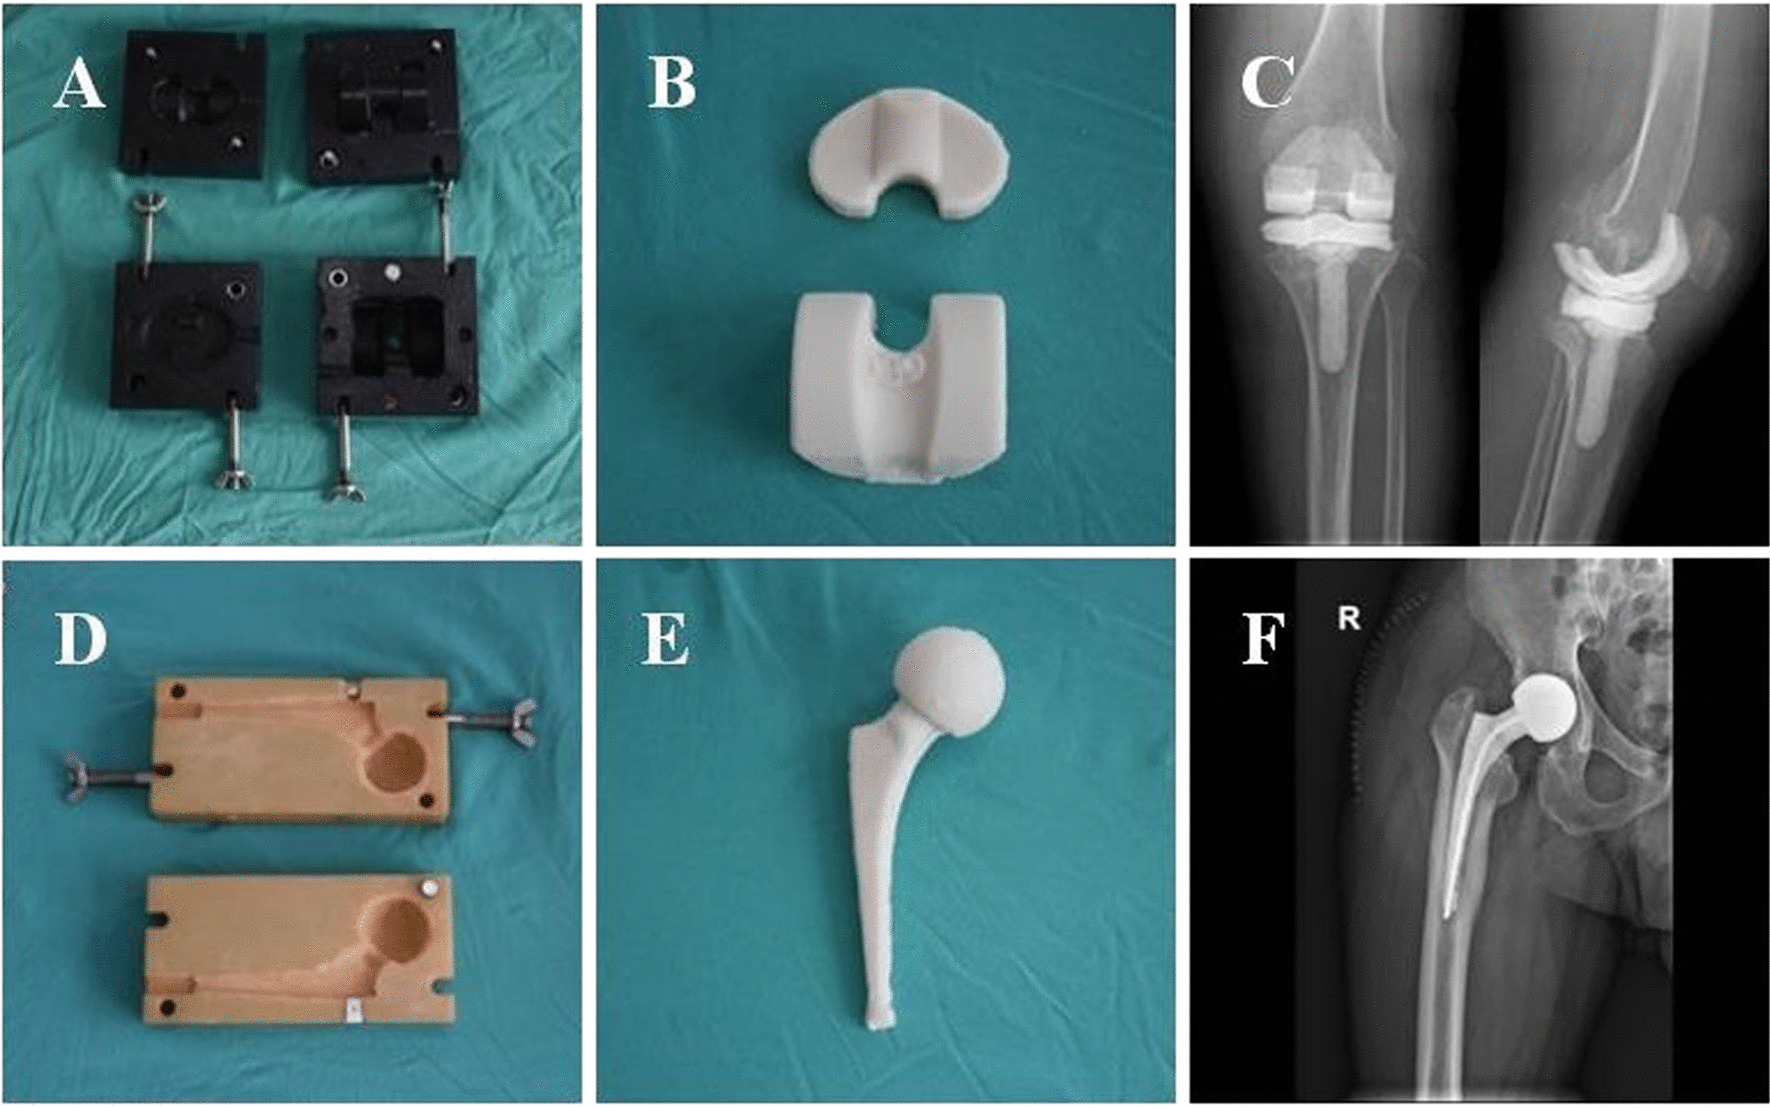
Fabrication of joint type antibiotic bone cement spacer. Self-made knee joint spacer mold imitating joint shape (A). Joint type knee antibiotic cement spacer made from mold (B). Anteroposterior and lateral radiographs of the left knee joint after spacer implantation (C). Self-made hip joint spacer mold imitating joint shape (D). Joint type hip antibiotic cement spacer made from mold (E). Anteroposterior radiograph of the right hip joint after spacer implantation (F)
